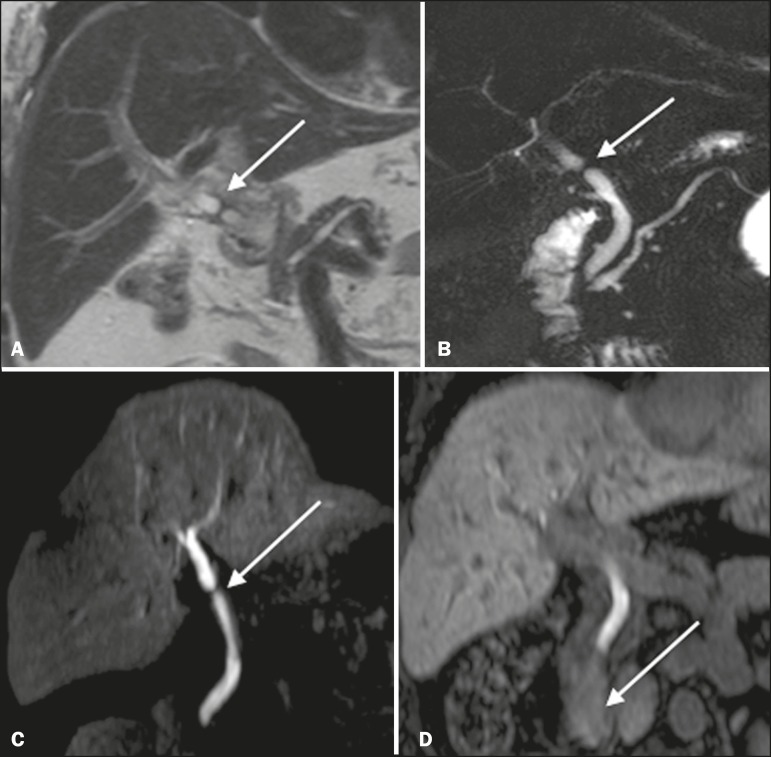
Stenosis of the biliary anastomosis after liver transplantation. Coronal T2-MRC and T1-weighted MRC (A and B, respectively): stenosis of the biliary anastomosis with mild dilatation of the intrahepatic biliary tree. Coronal fMRC at 40 min after the injection of gadoxetic acid (C,D): stenosis (C) and a delay in the progression of contrast to the duodenal arc (D). Obvious stenosis with a delay in the progression of the contrast medium through the biliary tract.
